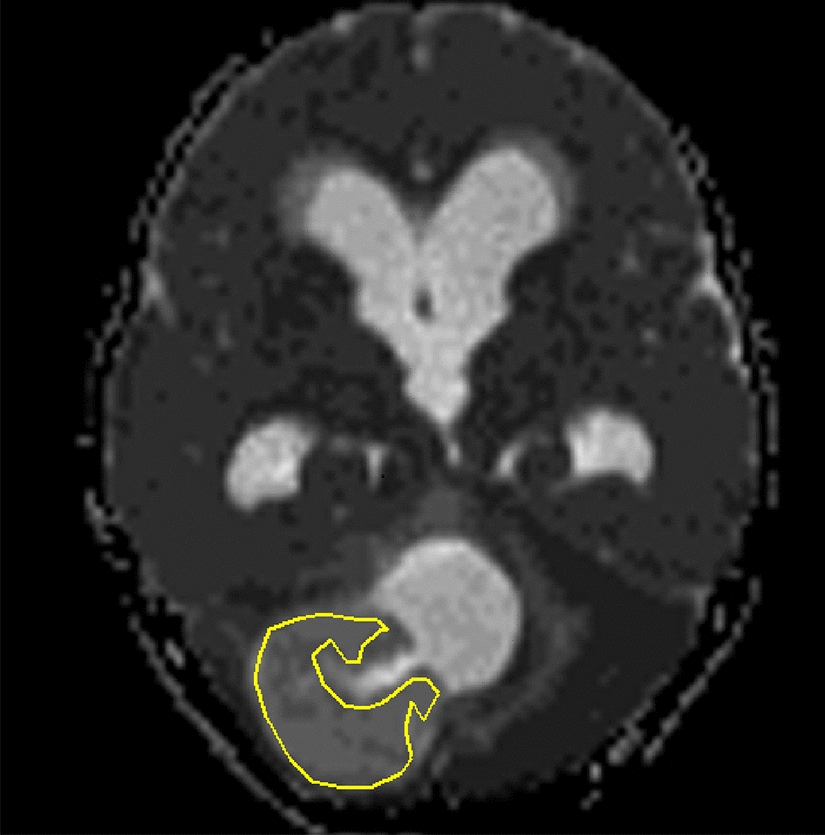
MRI ADC brain image of a 14-year-old female patient diagnosed with pilocytic astrocytoma which was radiologically and histo-pathologically identified as a benign tumor. The tumor area is surrounded by the ROI. The texture features were extracted form the selected area
